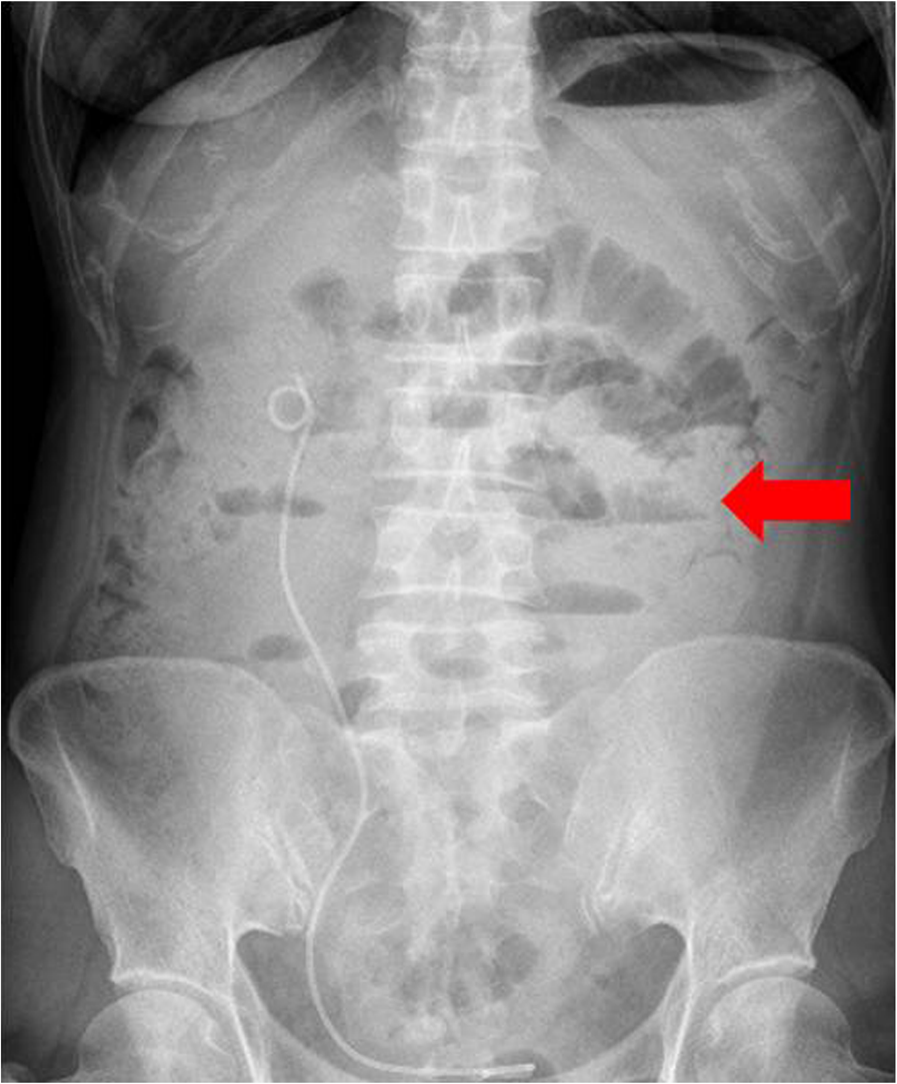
Abdominal plain film of incomplete intestinal obstruction. Notes: progressive disease after using Olaparib
